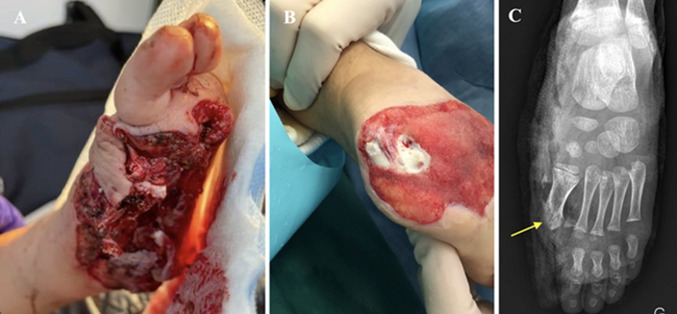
initial trauma: A) severe damage with soft tissue defect of the plantar, medial, and posterior sides of the left foot; B) bone exposure of the calcaneus after negative pressure wound therapy; C) frontal X-ray view of the foot showing trans-metatarsal amputation of the hallux with bony exposure of the head of the first metatarsal (yellow arrow)
